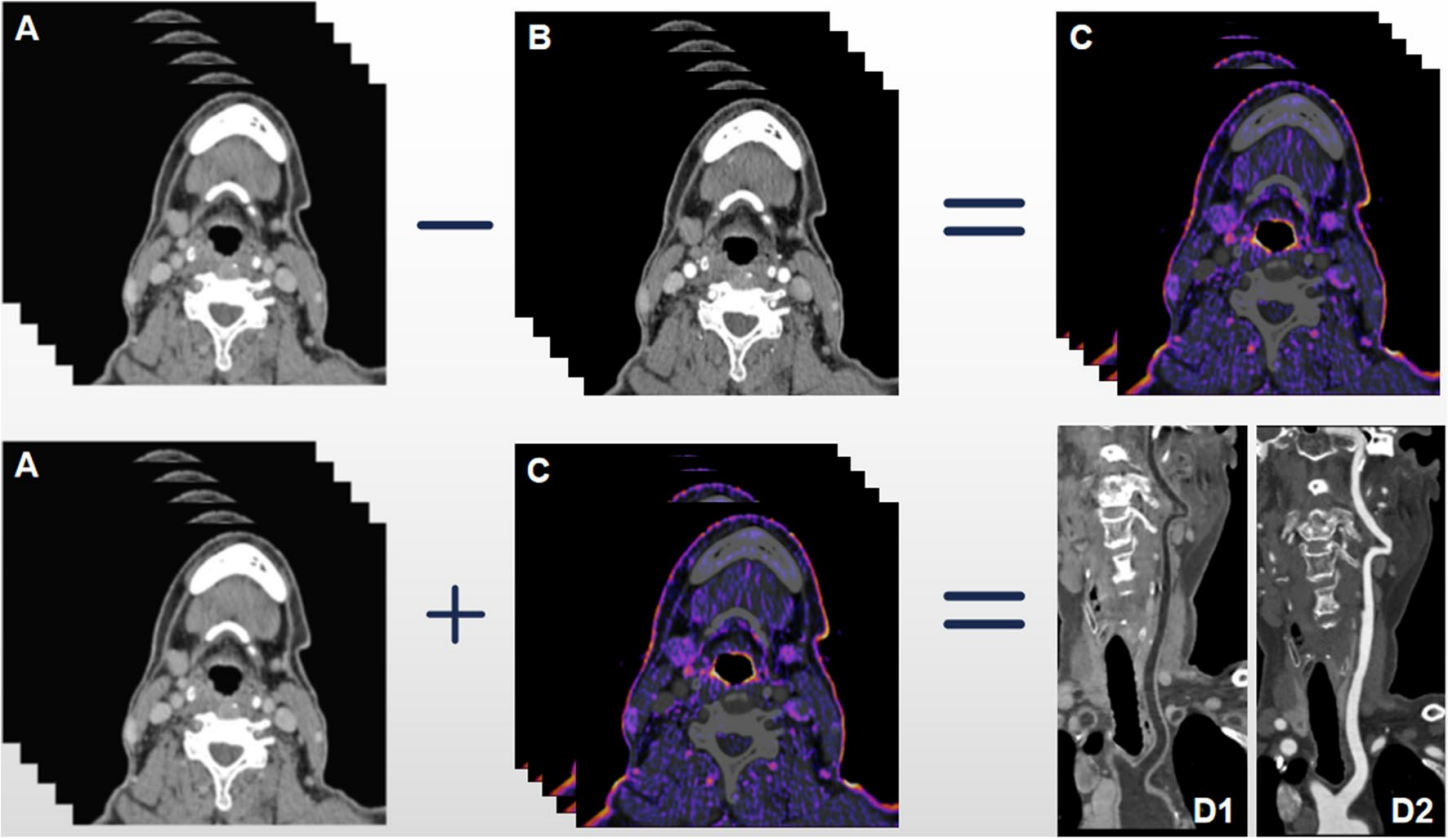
The principle of BBCT technique. Iodine mappings (C) were obtained by subtracting arterial-phase CT images (B) from the delayed-phase images (A). BBCT (D1) were obtained by adding delayed-phase images to iodine mappings, which displayed artery wall clearly. Conventional CTA (D2) poorly displayed with this WL/WW settings
